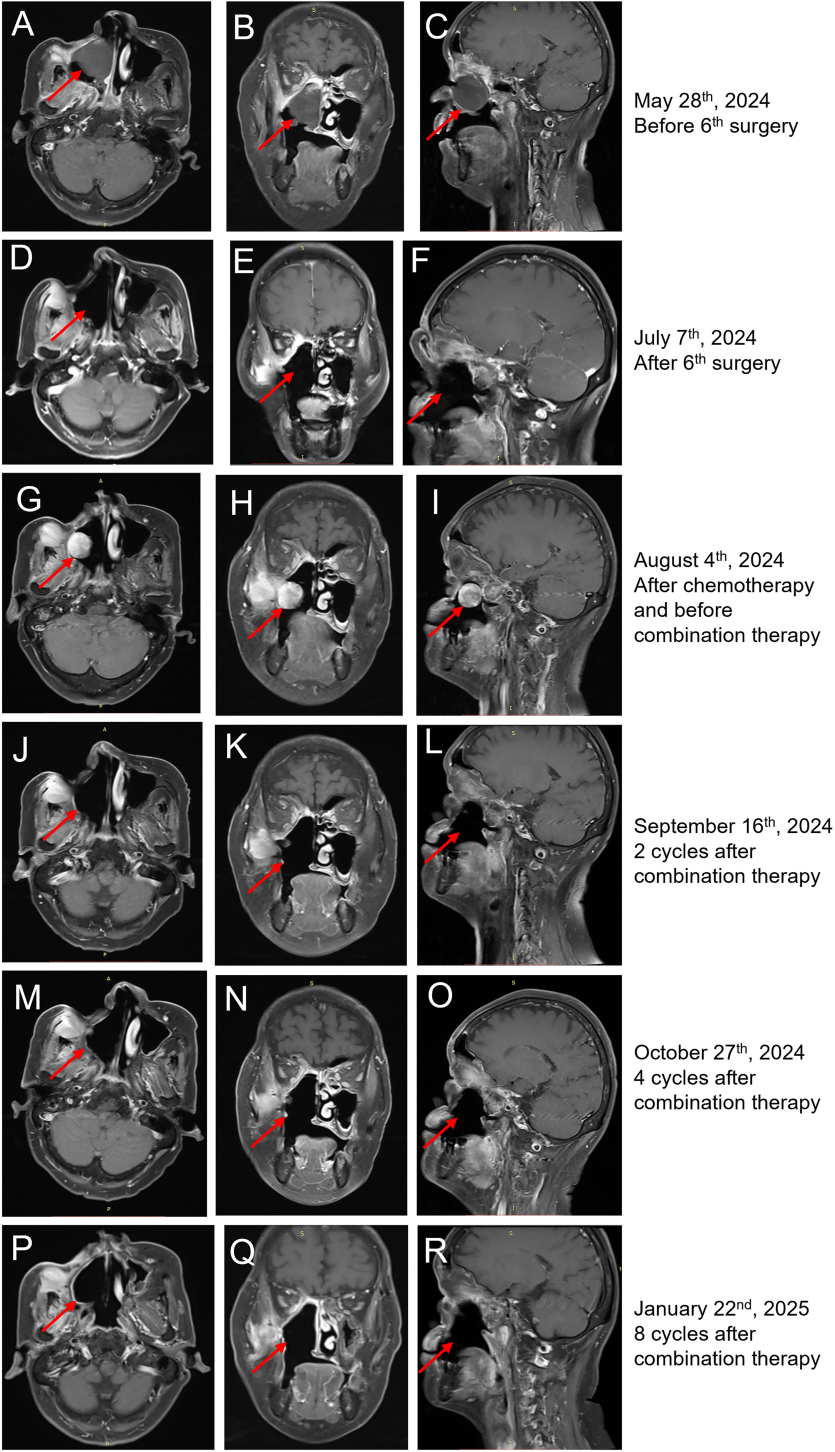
MRI findings of the case. (A–C) Horizontal (A), coronal (B), and sagittal (C) MRI of sinus before the sixth surgery on 28 May 2024. The tumor is indicated by red arrows. (D–F) Horizontal (D), coronal (E), and sagittal (F) MRI of sinus 1 month after the sixth surgery on 7 July 2024. (G–I) Horizontal (G), coronal (H), and sagittal (I) MRI of sinus before toripalimab + anlotinib treatment showed that the right nasal cavity, sinus, right maxillary sinus, turbinate, and palate displayed postoperative changes. The maxillofacial area was sunken, and the adjacent soft tissues were significantly enhanced. The right temporalis muscle and internal and external pterygoid muscles were thickening, and the signal was increased. There were strips of significantly enhanced shadows in the intermuscular space, which were dumbbell-shaped from the lateral wall of the upper maxillary sinus to the sinus cavity. The size is approximately 50 × 25 × 27 mm. The tumor is indicated by red arrows. (J–L) Horizontal (J), coronal (K), and sagittal (L) MRI of sinus after two cycles of toripalimab + anlotinib treatment showed reduced tumor lesions. (M–O) Horizontal (M), coronal (N), and sagittal (O) MRI of sinus after four cycles of toripalimab + anlotinib treatment showed continuous partial response. (P–R) Horizontal (P), coronal (Q), and sagittal (R) of sinus after eight cycles of toripalimab + anlotinib treatment showed continuous partial response.
